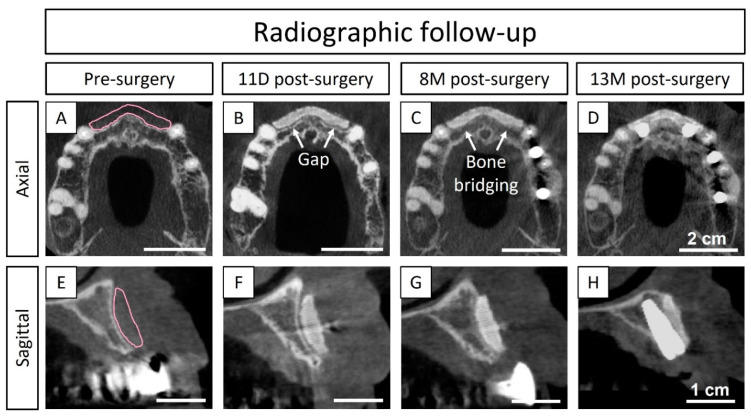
CBCT images acquired pre-surgery, 11 days, 8 months, and 13 months post-surgery (3 months after reentry and dental implant placement). (A–D) Axial projection; (E–H) sagittal projection, used for bone thickness measurements: (A,E) pink lines indicating the pre-designed bone graft and planned bone augmentation, (B,F) revealing a gap between the bone graft and the pristine bone, (C,G) demonstrating new bone ingrowth and bridging of the previous gap, (D,H) dental implant position after successful bone augmentation and implant placement.
